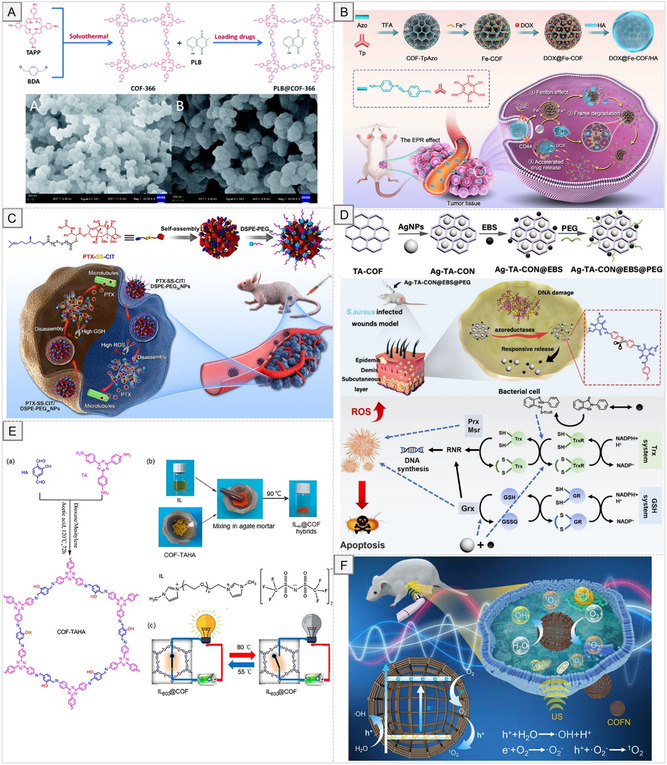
Stimuli‐responsive COFs. (A) pH‐responsive COFs. Reproduced with permission from ref. [120]. Copyright 2022 Royal Society of Chemistry. (B) Redox‐responsive COFs. Reproduced with permission from ref. [119]. Copyright 2023 Royal Society of Chemistry. (C) Redox‐responsive COFs. Reproduced with permission from ref. [122]. Copyright 2018 American Chemical Society. (D) Enzyme‐responsive COFs. Reproduced with permission from ref. [123] Copyright 2022 American Chemical Society. (E) Thermo‐responsive COFs. Reproduced with permission from ref. [124]. Copyright 2023 American Chemical Society. (F) Ultrasound‐responsive COFs. Reproduced with permission from ref. [125]. Copyright 2023 American Chemical Society.
